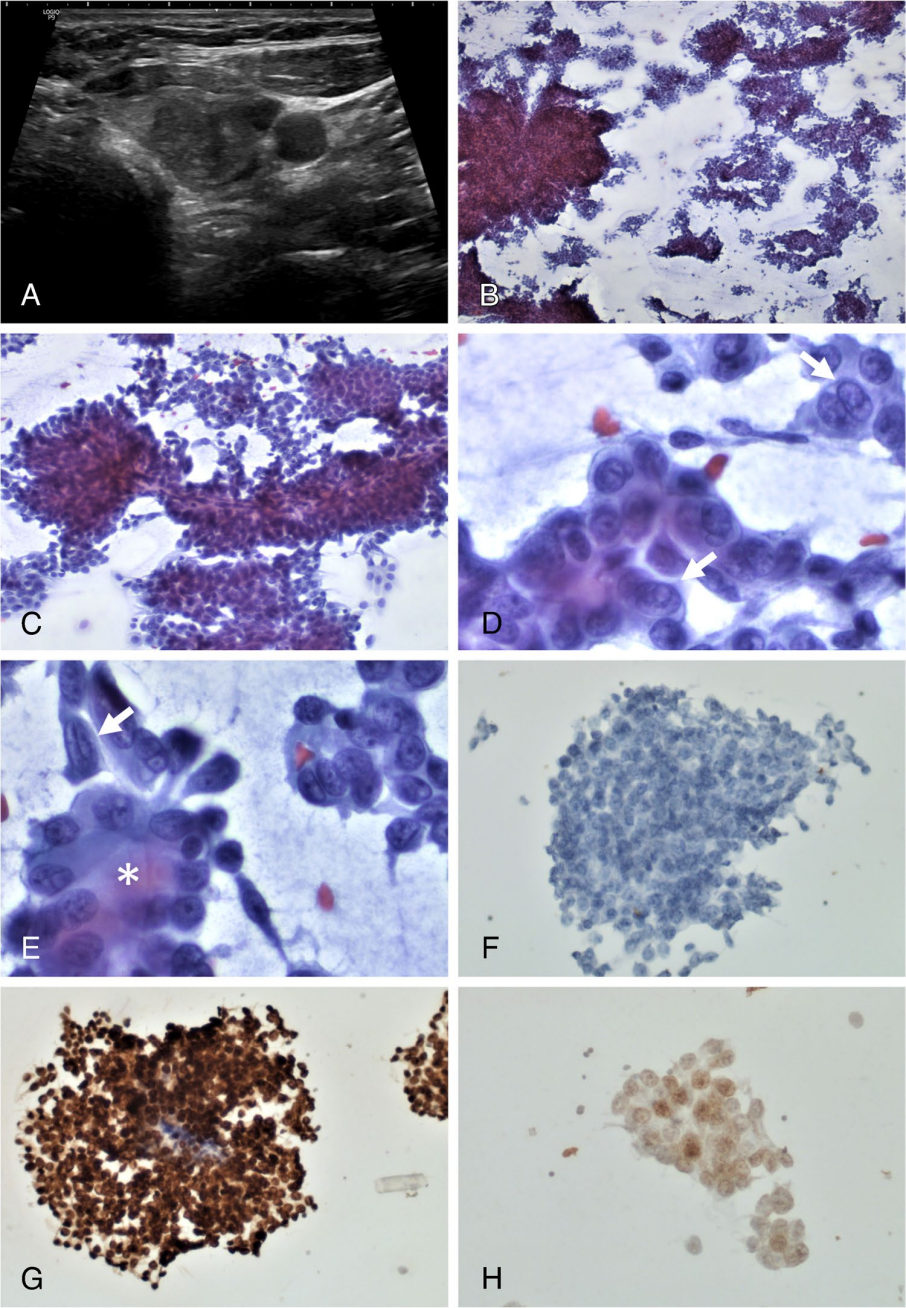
Ultrasound image showing a predominantly solid, hypoechoic thyroid nodule (A). The cytological smear is highly cellular and composed of monolayer sheets and papillary-like fragments (B and C). At a higher magnification, intranuclear cytoplasmic pseudoinclusions (D, arrows) and nuclear grooves can be seen (E, arrow). Follicular structures can also be detected (E, asterisk). Tumor cells (on cell block preparations) are negative for thyroglobulin (F) but strongly positive for TTF1 (G) and weakly positive for PAX8 (H)
